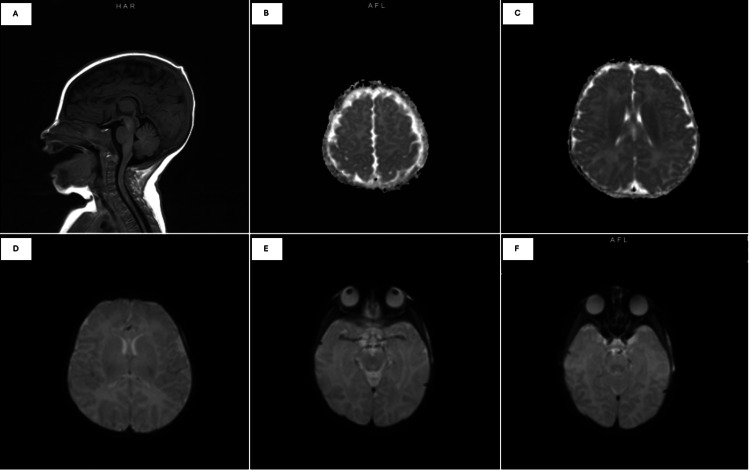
MRI brain without contrast MRI: magnetic resonance imaging; DWI: diffusion-weighted imaging (A) Sagittal T1-weighted image demonstrating normal brain anatomy without structural abnormalities. (B-C) Axial T2-weighted images showing symmetric cerebral hemispheres without focal lesions. (D) DWI without evidence of restricted diffusion. (E-F) Additional axial sequences demonstrating no evidence of hypoxic-ischemic injury or intracranial pathology
